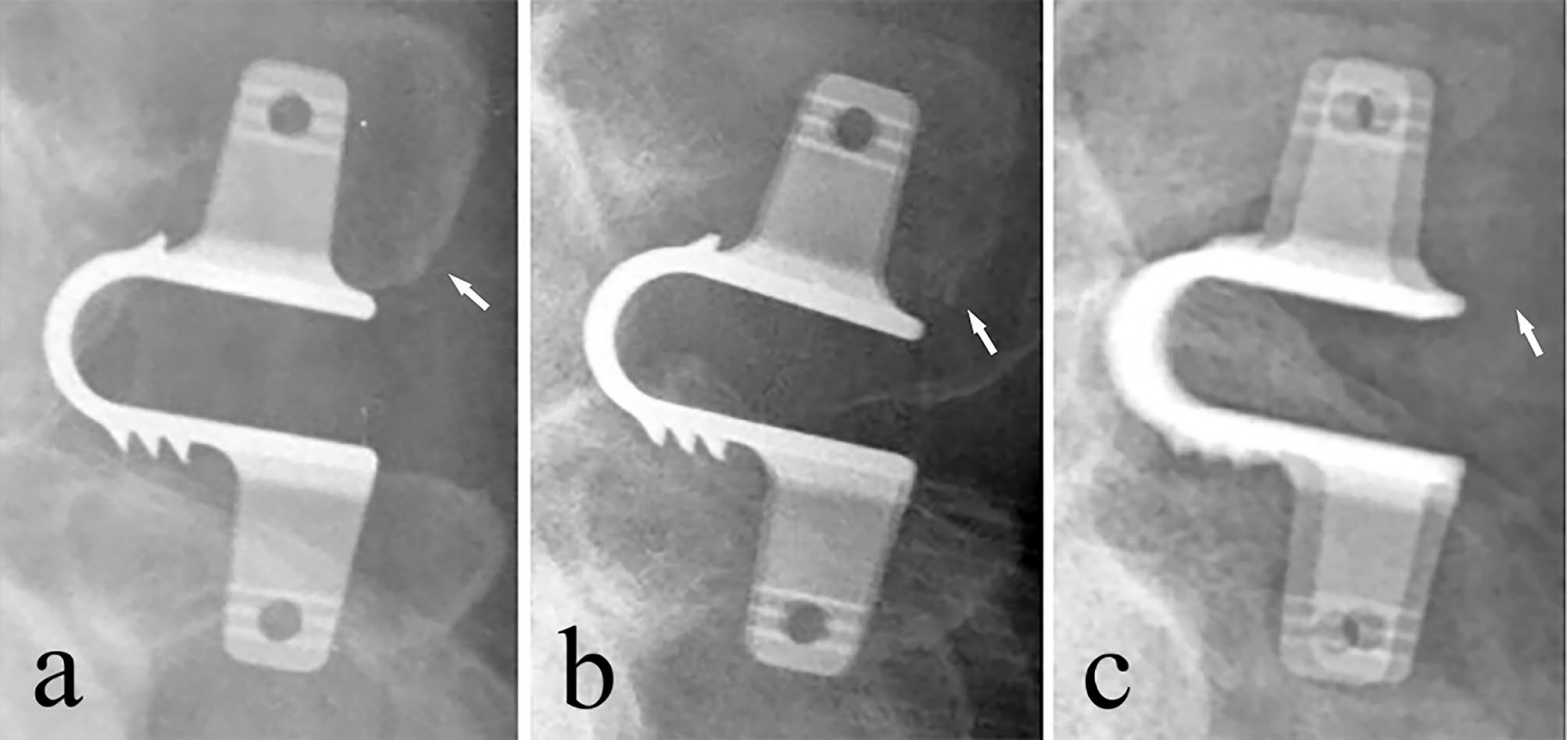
Radiographs of one patient, lateral views. a: Preoperative image. b: Postoperative image at one-year. c: Postoperative image at last follow-up. Arrows indicate osteolysis
